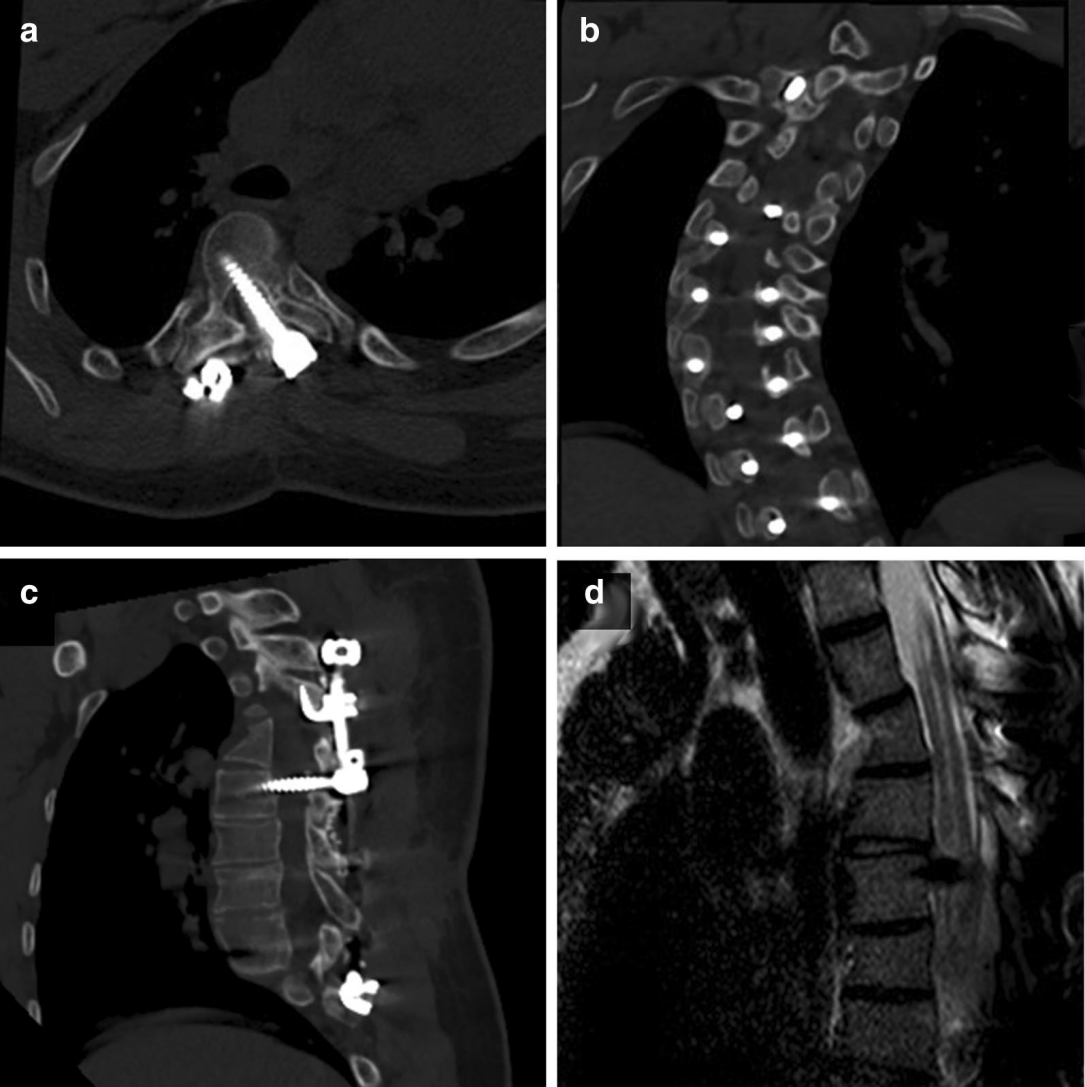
CT scan showing the gross misplacement of the pedicle screw, which crossed the center of the vertebral canal on axial (a), coronal (b) and sagittal (c) planes. d Same sagittal plane of spinal MRI showing an increased diameter of the syrinx adjacent to a left T5 pedicle screw crossing the spinal canal
